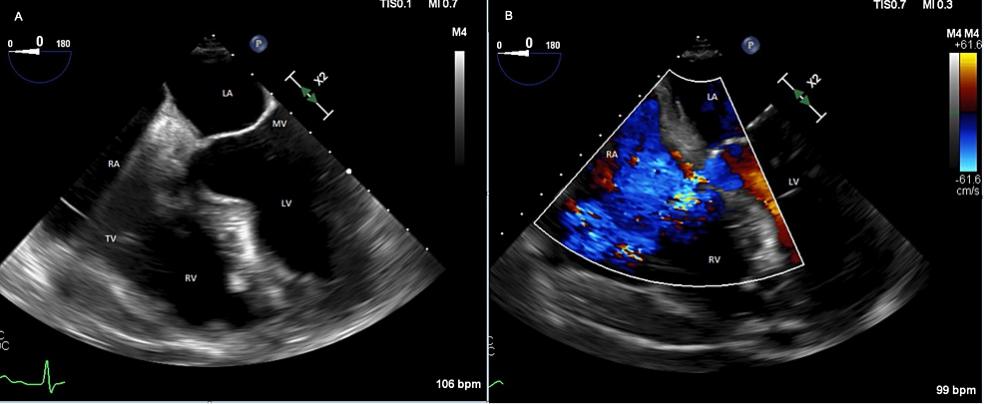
Transesophageal view of the Gerbode defect. Panel A is a four-chamber view with the noted Gerbode defect superior to the tricuspid valve. Panel B with Doppler color wave through the Gerbode defect, from the left ventricle into the right atrium.
